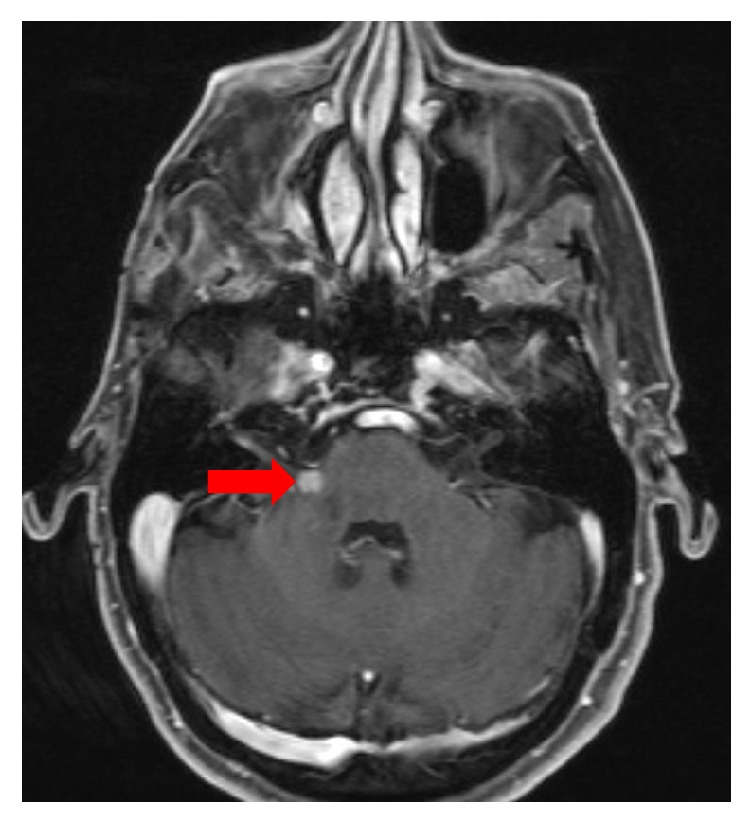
T1-weighted image with contrast, highlighting new right-sided cerebellopontine angle lesion (arrow).
